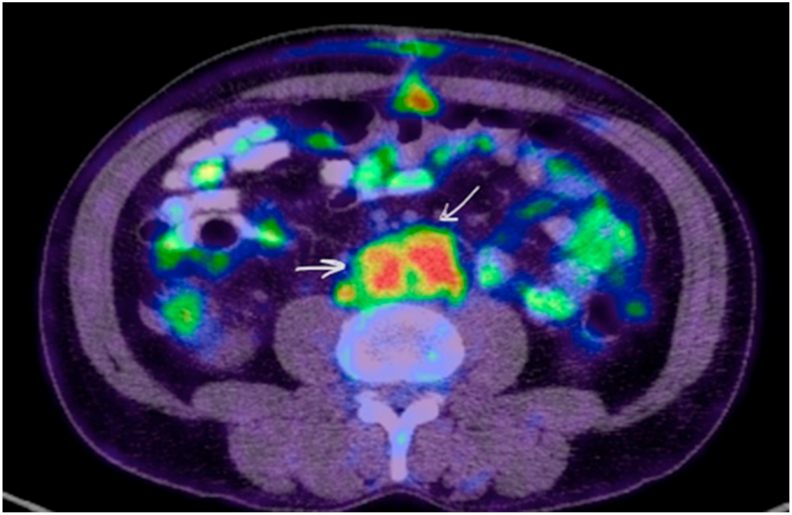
FDG Whole body PET CT showing Retroperitoneal mass lesion encasing the aorta, inferior vena cava and common iliac arteries with hypermetabolic activity (SUV max 9.1). (This figure does not need to be printed in color). (For interpretation of the references to color in this figure legend, the reader is referred to the Web version of this article.)
